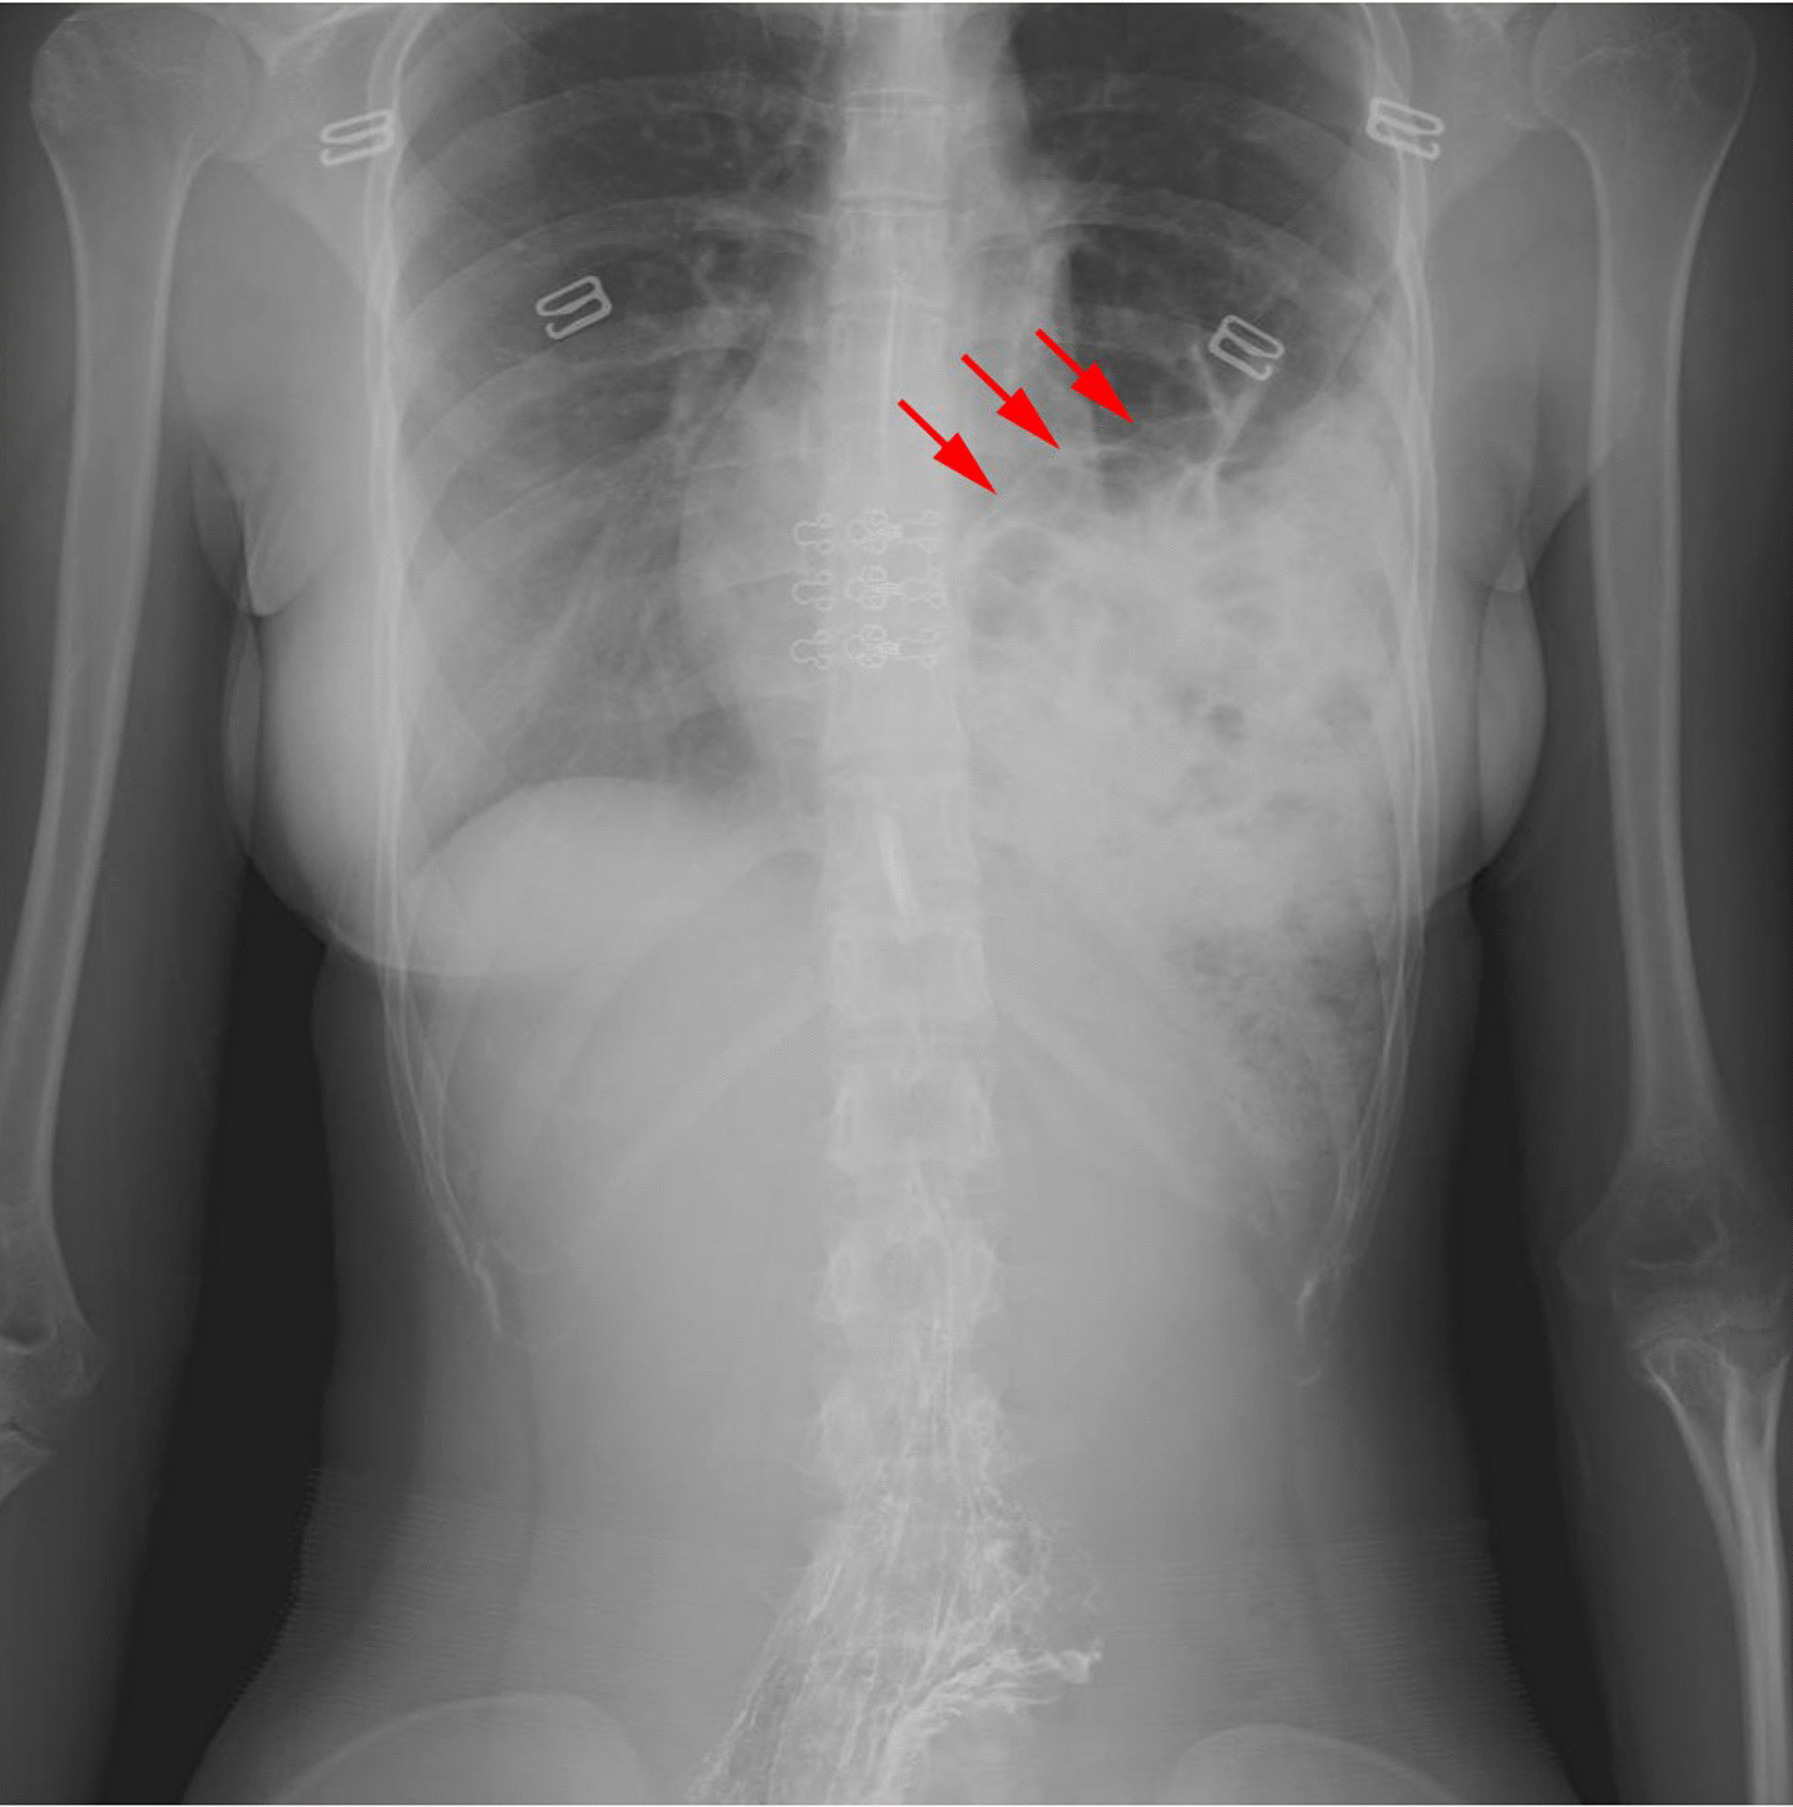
An X-ray showing herniated loops in the left hemithorax, which appeared as multiple lucent shadows and severely reduced lung space (arrow)
